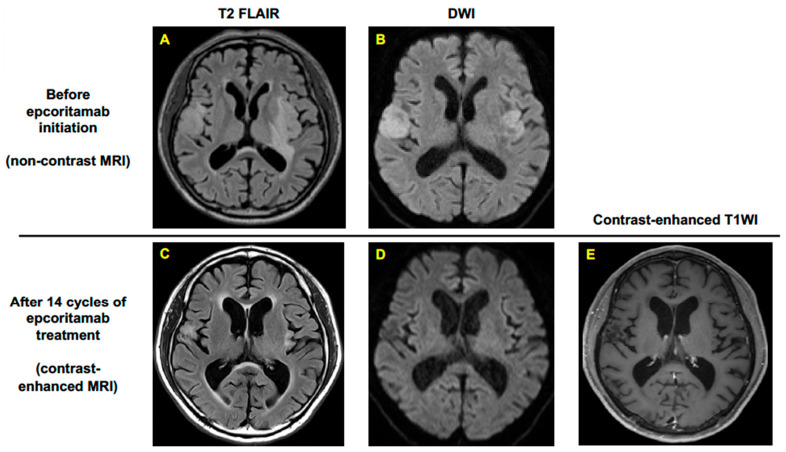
Brain magnetic resonance imaging before initiation of epcoritamab and after 14 cycles of treatment in a patient with clinically and radiologically suspected CNS relapse of DLBCL. Pretreatment non-contrast brain MRI demonstrated multiple intracranial lesions on T2 FLAIR imaging (A) and diffusion-weighted imaging (B). After 14 cycles of epcoritamab treatment, follow-up MRI showed resolution of the previously identified lesions and surrounding edema on T2 FLAIR imaging (C) and diffusion-weighted imaging (D). Post-treatment contrast-enhanced T1-weighted imaging (E) showed no obvious enhancing residual lesion, consistent with radiological CR. Because only non-contrast MRI was performed immediately before epcoritamab initiation, matched pre- and post-treatment contrast-enhanced T1-weighted images were not available. Representative axial images at approximately corresponding anatomical levels are shown. CNS, central nervous system; DLBCL, diffuse large B-cell lymphoma; DWI, diffusion-weighted imaging; FLAIR, fluid-attenuated inversion recovery; MRI, magnetic resonance imaging.
